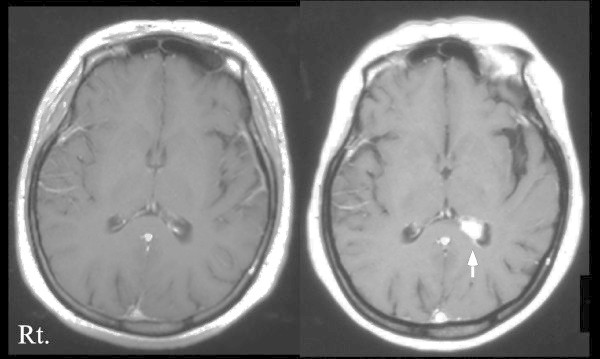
Contrast T1-weighted magnetic resonance images (MRI). Initial MRI showed no abnormal enhancement (left panel), but follow-up MRI revealed enhancement of the choroid plexus and posterior horn of the lateral ventricle, with no ventricular enlargement (right panel).
